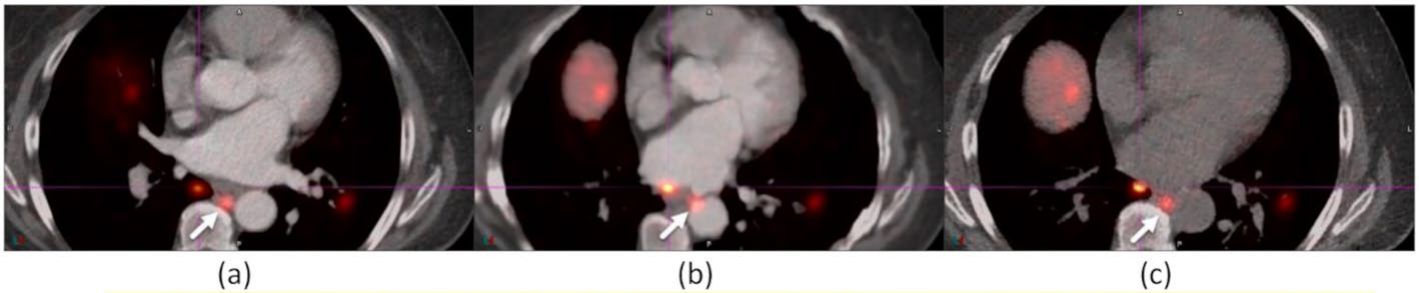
A 0.7-cm lung lesion at the crosshair in baseline (a) and DDG CT (c) was mispositioned to the left atrium of the heart in AIR (b). A separate lymph node (indicated by an arrow) by the vertebral body and the aorta in baseline and DDG CT was intruding the aorta, which was no longer circular in AIR. The SUV_max_ values of the lung lesion were 20.3 for baseline, 25.0 for AIR, and 22.6 for DDG CT
